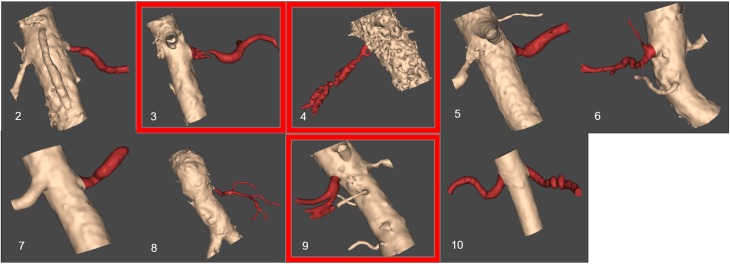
Resulting segmented patient renal geometries. Segmented volumes of renal arteries: unsuccessful segmentation examples based on the provided CT images are indicated by a red frame. The number for each image corresponds to patient number in Table 1.
